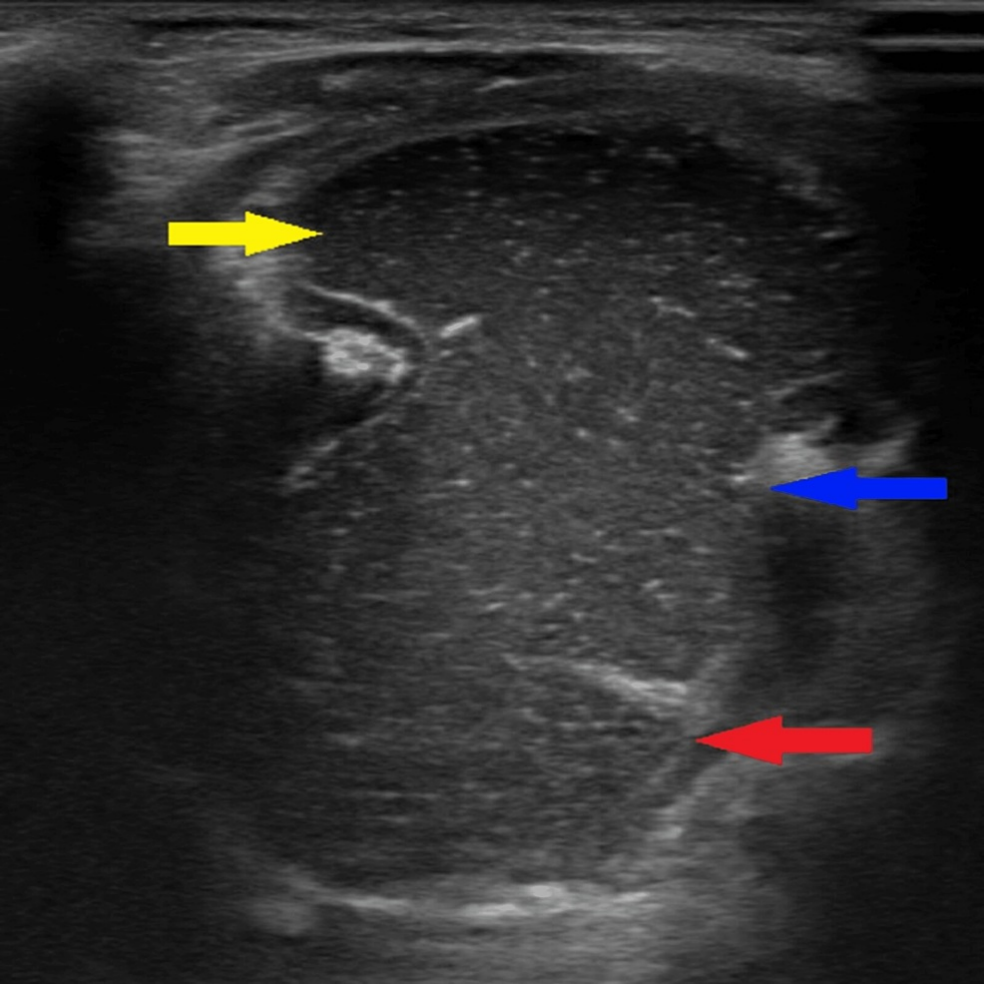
B-mode ultrasound in the oblique sagittal plane showing a sublingual component (yellow arrow) and a submandibular component (red arrow). A defect in the mylohyoid muscle is present through which the ranula is extending from the sublingual to submandibular region (blue arrow).
